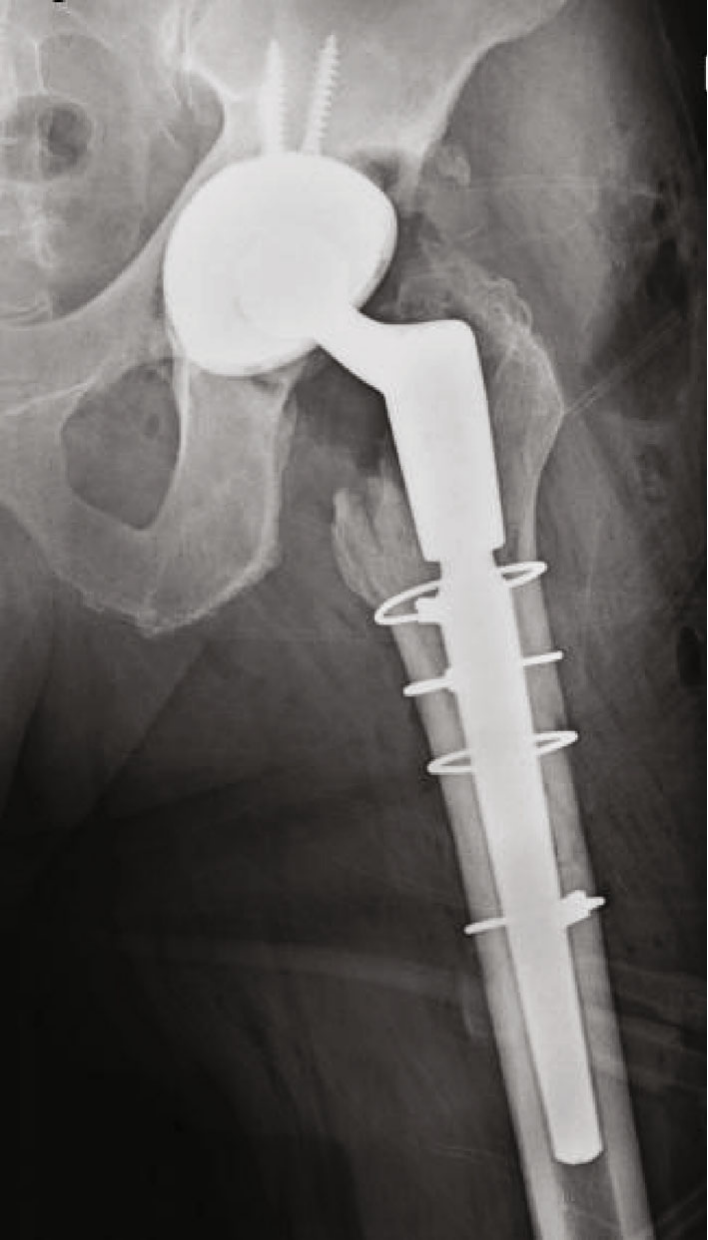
Intraoperative radiograph following successful reduction and component revision.
